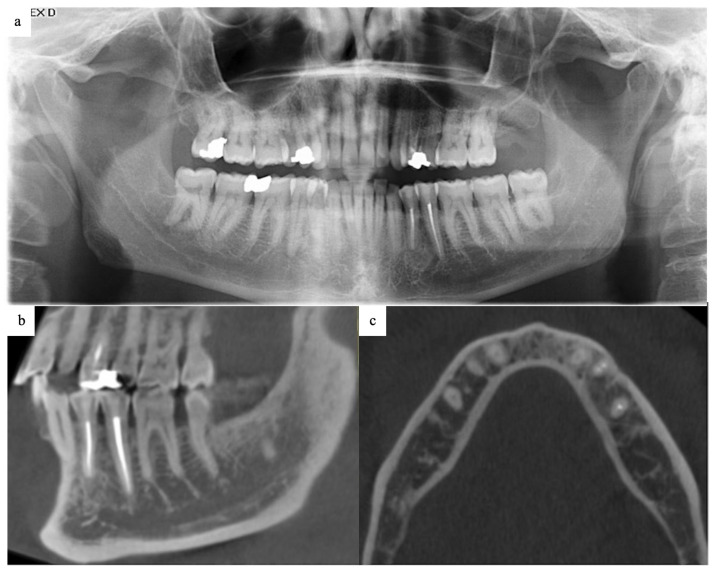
(a) Full-mouth OPG taken 18 months after the surgery, showing normal bone regeneration at the site of the enucleated lesion. (b,c) CBCT taken 36 months after the surgery, showing uneventful bone healing of the cystic bone defect without evidence of lesion recurrence.
